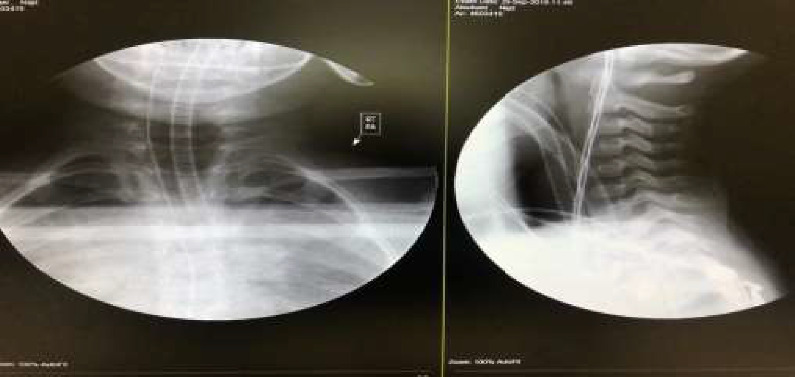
Intraoperative cervical X-ray showing complete removal of the foreign body.
